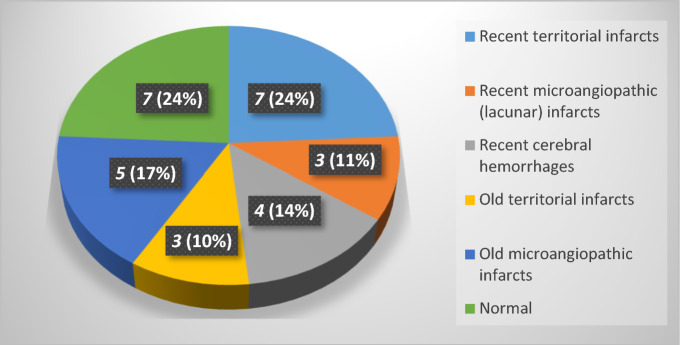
The distribution of patients, revealing brain MRI findings, in RT-PCR proved SARS-CoV-2 pneumonia, and presented with focal neurological manifestations.
